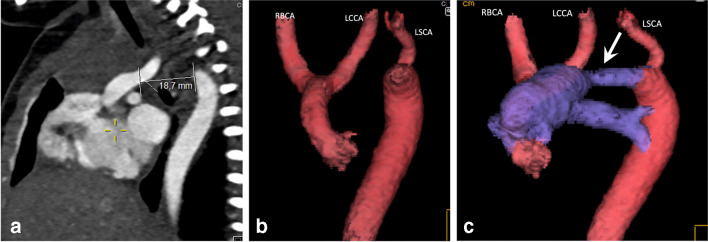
Contrast-enhanced computed tomography angiography images in a newborn boy with circulatory collapse on day one of life, in whom echocardiography showed poor quality views of the aortic arch. a Sagittal contrast-enhanced image shows a long gap (18.7 mm) between the interrupted aortic arch and the descending aorta. b, c Anteroposterior (b) and sagittal oblique (c) volume rendered images with the ascending and descending aorta and branches in red and pulmonary artery, left pulmonary artery, and open arterial duct (*arrow in*
